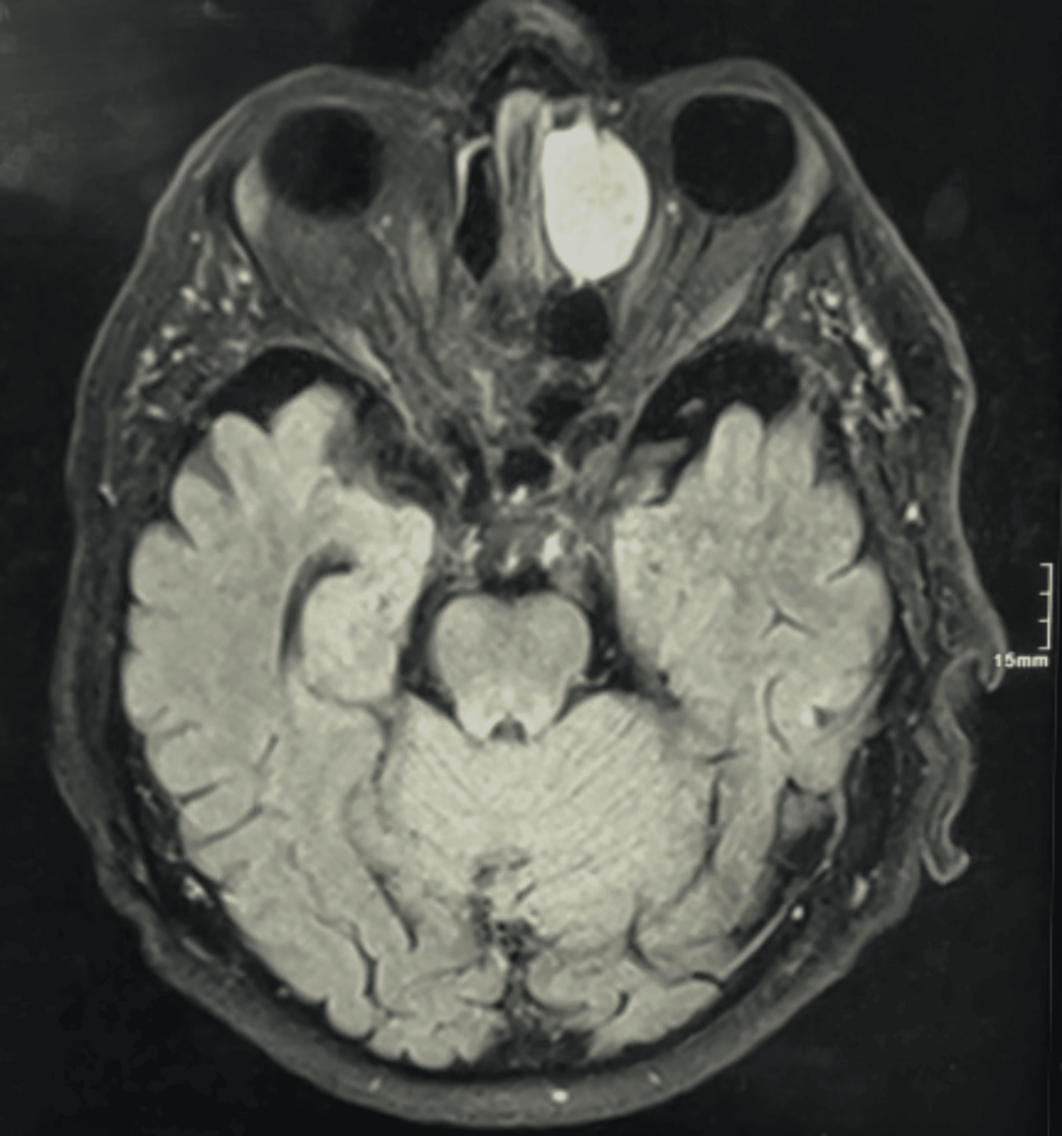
Preoperative cerebral MRI A 27 x 26 x 19 mm nodular lesion centered on the left anterior ethmoid air cells, showing a bulgy appearance with underlying bone lysis and compressing over the medial wall of the left orbit, and abutting the medial rectus muscle with no evidence of detectable neoplastic lesion with IV contrast. MRI: magnetic resonance imaging, IV: intravenous
